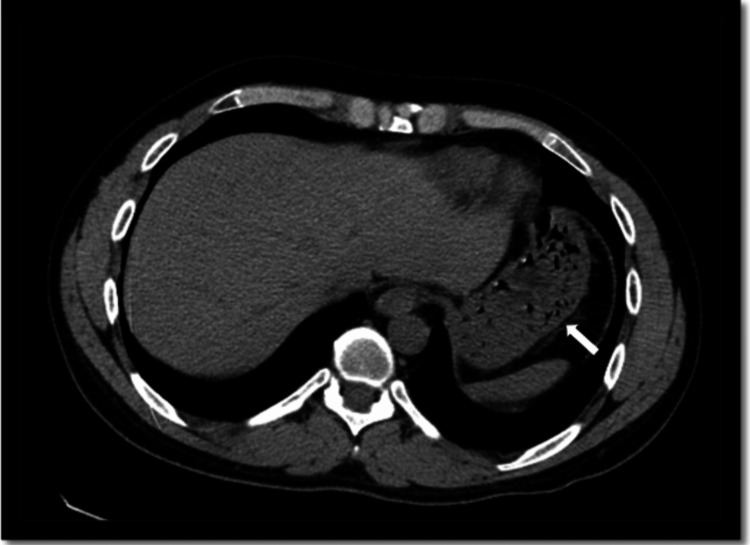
Axial view of CT abdomen and pelvis without contrast Circumferential thickening of the gastric wall with multiple small collections of air within the gastric wall and mild peri-gastric fat stranding (white arrow points to intramural gas in the stomach).
